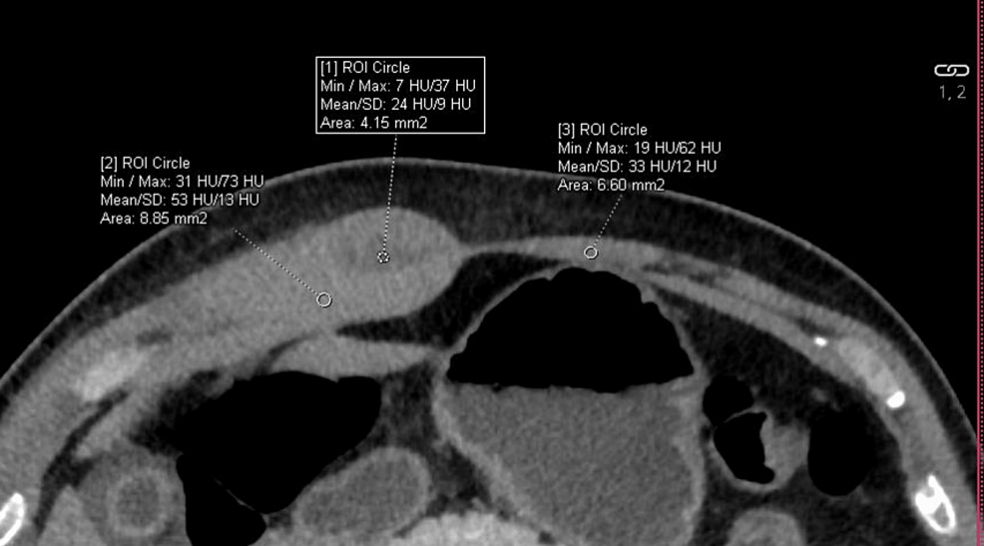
Right rectus sheath shows collection on CECT CECT: contrast-enhanced computed tomography
